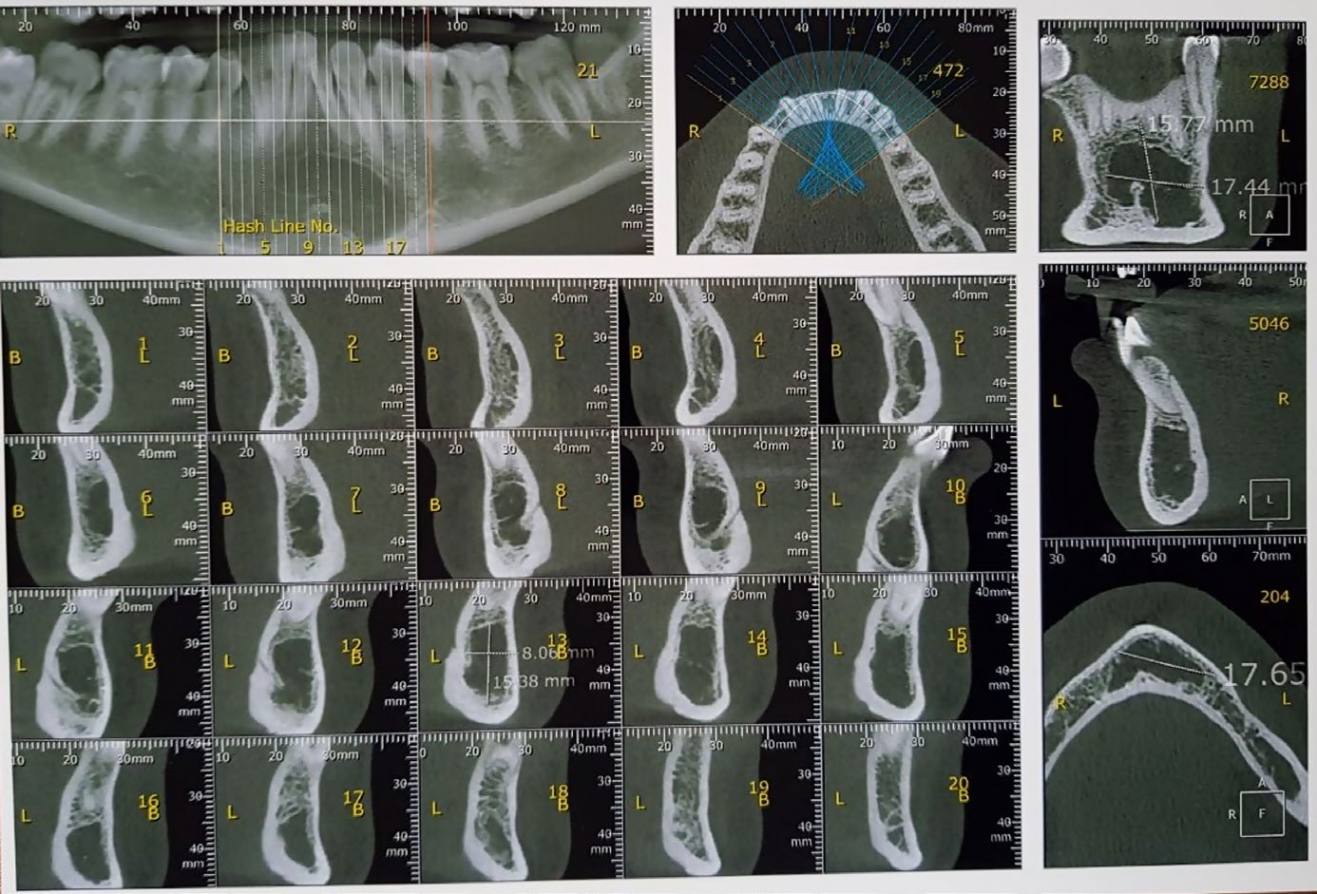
Radiograph of a patient with intraosseous lipoma. This is CBCT (cone beam computed tomography) view of the mandible, which shows a unilocular radiolucent lesion in the symphysis of the mandible
